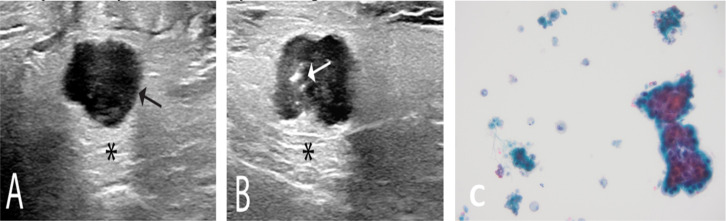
Ultrasonography of the right parotid gland and fine-needle aspiration cytology sample. Axial (A) and longitudinal (B) sonogram of the right parotid gland showing a single lobulated and well circumscribed hypoechogenic cystic lesion inside the superficial parenchyma (black arrow) with acoustic enhancement (black asterisk). The lesion present with few echogenic foci (white arrow) in the upper part that may represent debris or particles suspended in fluid. Liquid-based preparation of fine-needle aspiration of right intraparotid lesion (C) displaying pseudopapillary cluster in a cystic background with « dirty » necrosis and amorphous material (Papanicolau, 400x).
